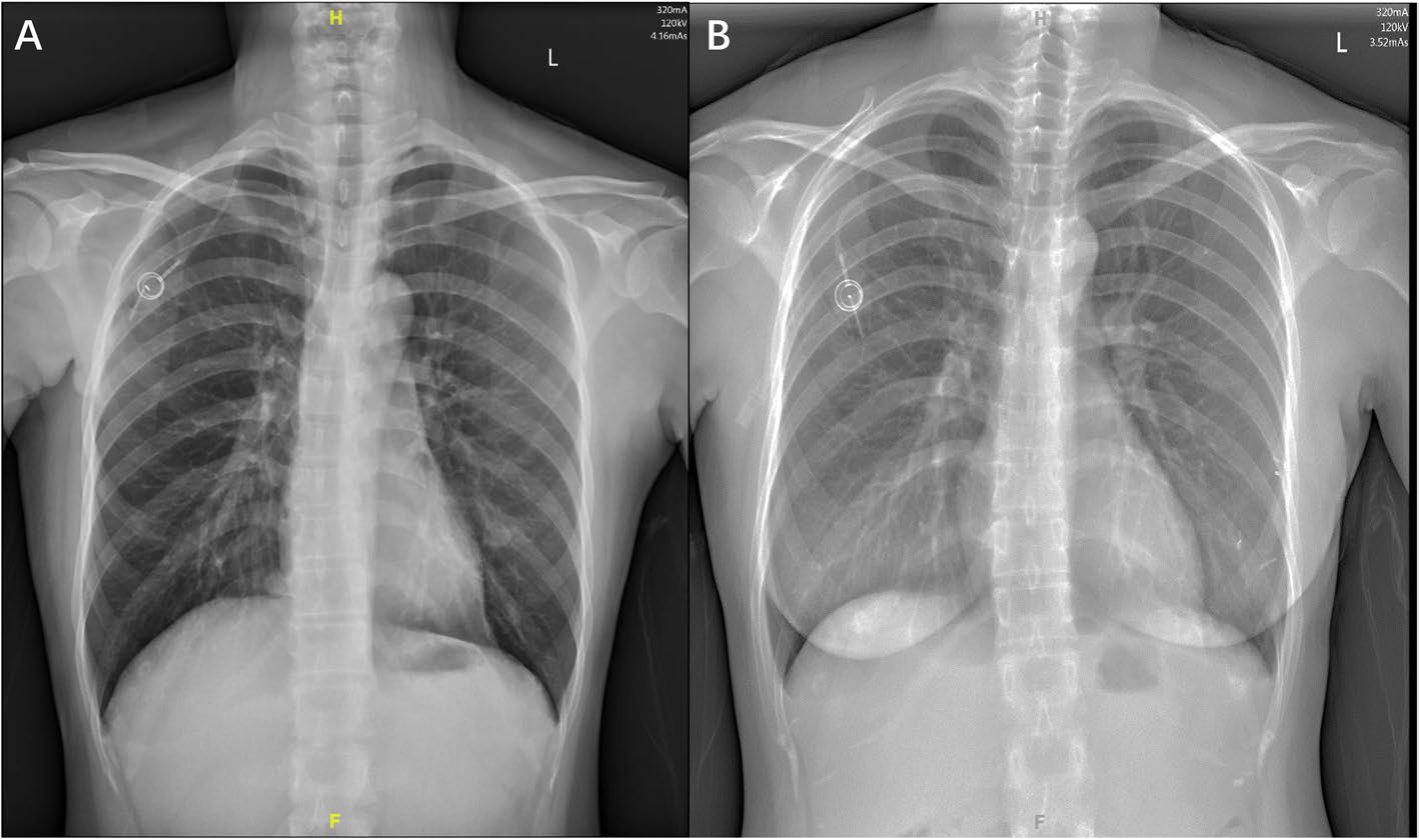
Chest X-rays of patients right after infusion ports implantation. A Chest X-ray of patient with CVC puncture through IJV. B Chest X-ray of patient with CVC puncture through AxV/SCV. CVC: Center Venous Catheter. IJV: Internal jugular vein. AxV/SCV: Axillary/Subclavian vein
